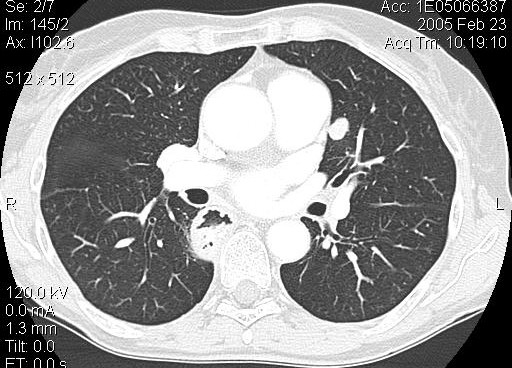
Computed tomography: well delimited pulmonary nodule of 13 mm in diameter of the LUL.
